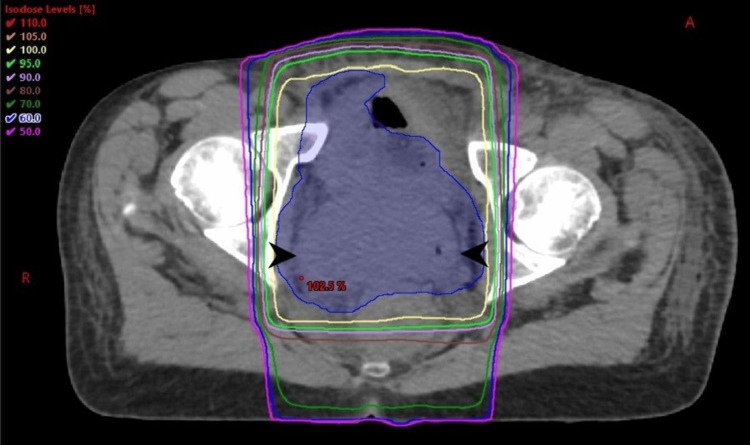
Axial CT planning scan of potential radiotherapy to control pain and bleeding in necrotic cervical mass Black arrowheads bracket cervical mass. The planning tumor volume (PTV) is indicated by blue-shaded volume. Radiation therapy was planned using a four-field 3D treatment technique. Isodose lines indicate the percentage of prescription radiation dose, as indicated by the legend at the top left.
